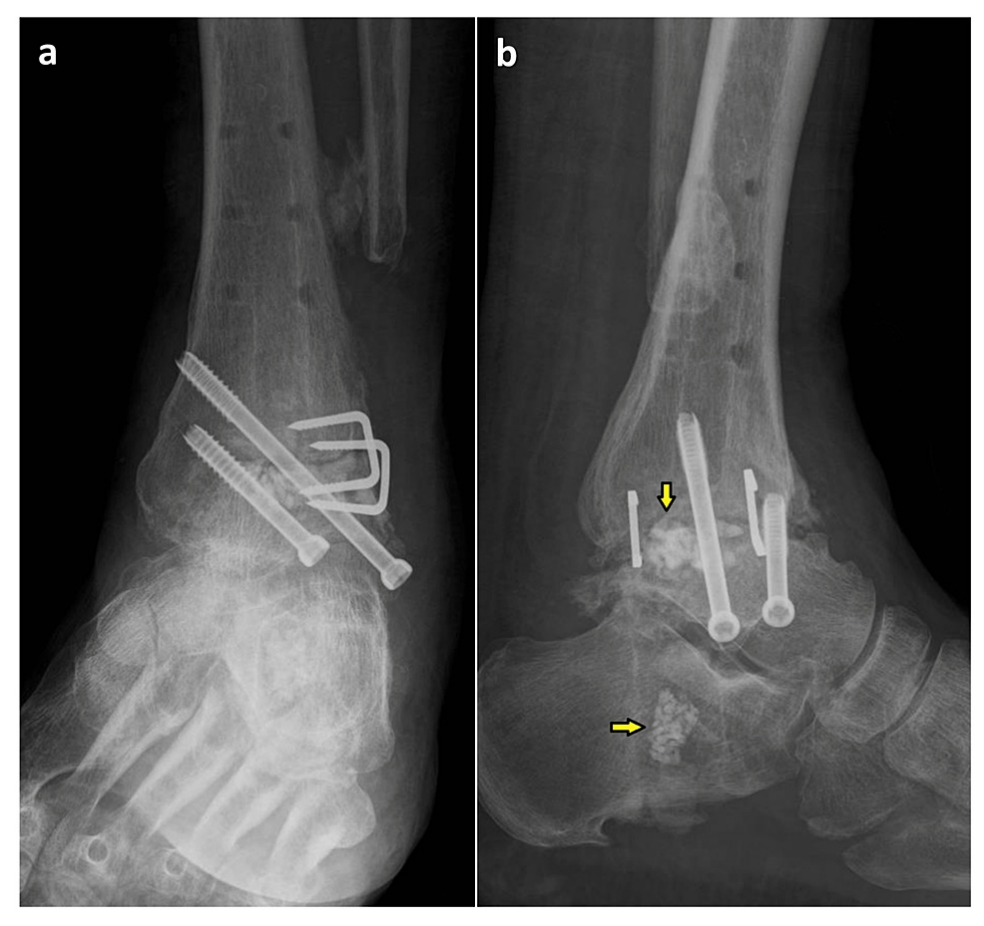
Plain radiographs just after surgery (a) anteroposterior and (b) lateral images
